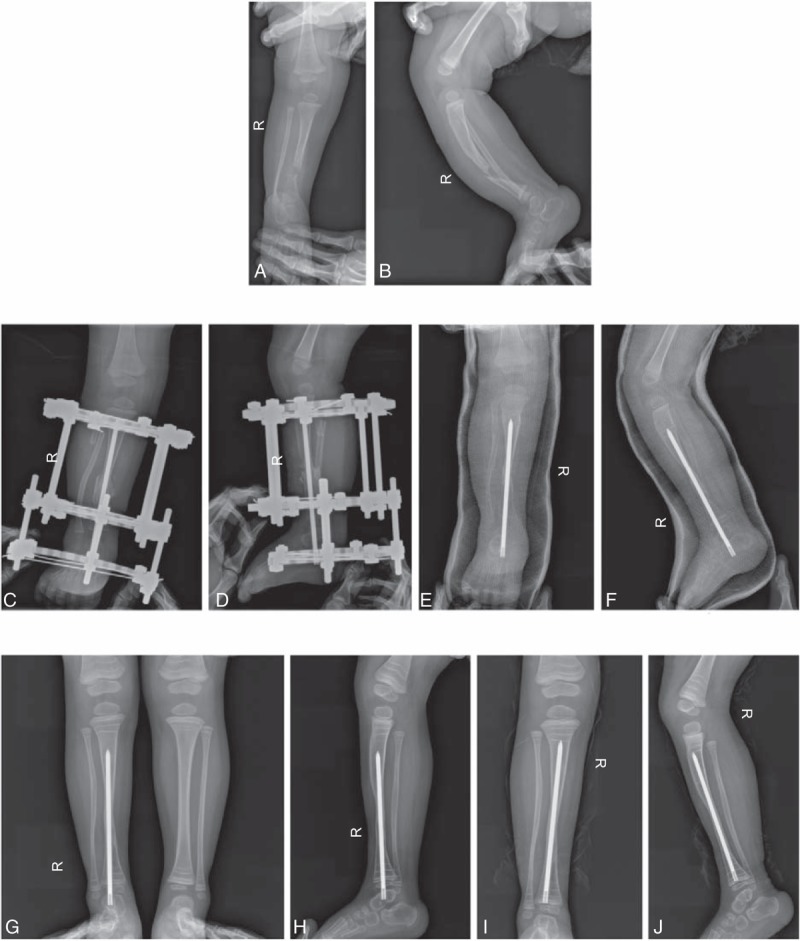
Typical case: Preoperative frontal (A) and lateral (B) radiographs of a 2-year-old boy, showing congenital pseudarthrosis of the right tibia and fibulae with neurofibromatosis type 1. Before removing of Ilizarov apparatus no any gaps existed between wrapped graft and previous pseudarthrosis of the tibia on frontal (C) and lateral (D) radiographs at 3 months after operation. Anteroposterior (E) and lateral (F) radiographs showed union of previous pseudarthrosis with evident spindle-shaped expansion in site of wrapping graft at 6 months after operation. Anteroposterior (G) and lateral (H) radiographs exhibited some remodeling of previous pseudarthrosis with reduced expansion in site of wrapping graft at 15 months after operation. Anteroposterior (I) and lateral (J) radiographs revealed continuous remodeling of previous pseudarthrosis with apparent increase of thickness and density of cortex in site of wrapping graft at 3 years following operation. The distal tip of rod had been pushed into distal epiphysis of the tibia because of precluding functional motion of the ankle joint.
